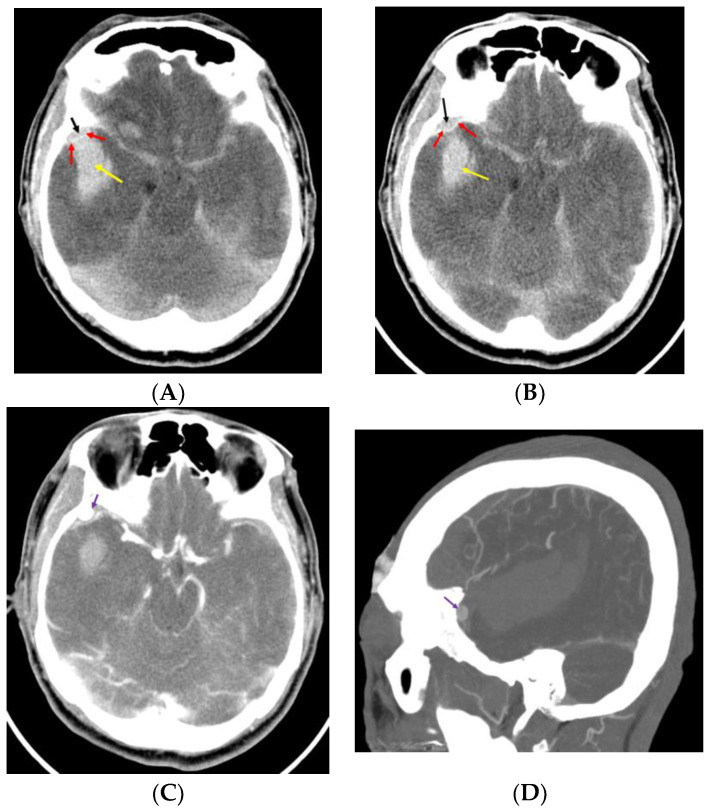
Preoperative computed tomography (CT) before (A,B) and after (C,D) contrast injection. Pre-contrast CTs demonstrate a small epidural hemorrhage (EDH) and intracerebral hemorrhage (ICH) (black arrow, EDH; red arrow, dura mater; yellow arrow, ICH). (A) ICH in contact with EDH. Post-contrast CTs (C,D) show a suspicious pseudoaneurysm located in the epidural space (violet arrow: suspicious pseudoaneurysm).
